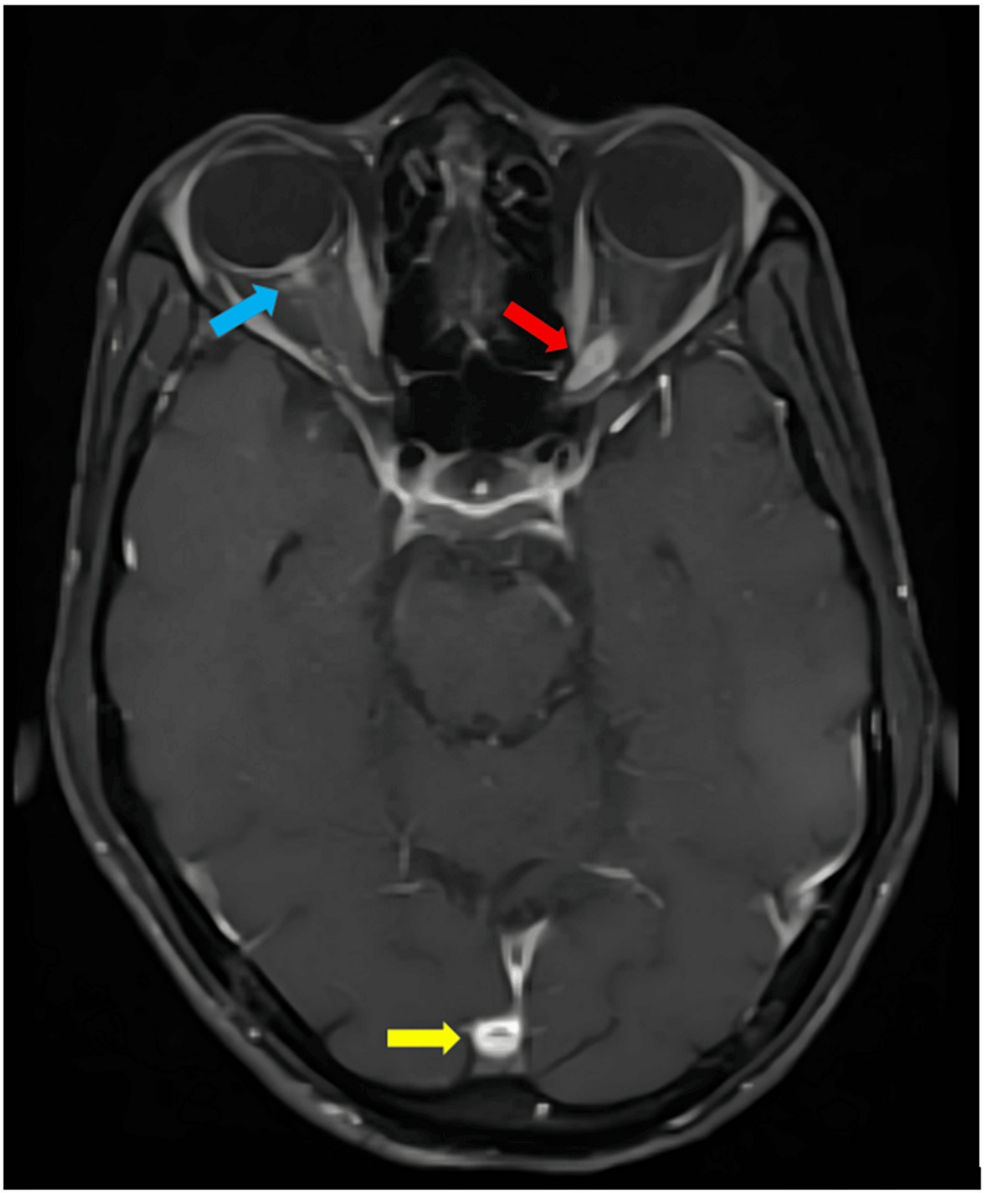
T1W contrast-enhanced MRI orbit Blue arrow: an ill-defined heterogenous enhancing lesion measuring approximately 4 × 2 mm was noted at the optic nerve head causing mild flattening of the posterior sclera; diffuse thickening of the optic nerve in the right optic canal was noted without any extension anterior to the globe. Red arrow: a well-defined heterogenous enhancing lesion measuring approximately 6× 3 mm was noted in the left optic nerve at the apex of the orbit; diffuse thickening with buckling of the optic nerve in the optic canal was noted without any extension beyond the optic canal. Yellow arrow: evidence of a ring-enhancing lesion was noted in the medial right occipital lobe parenchyma almost in the midline MRI: magnetic resonance imaging
